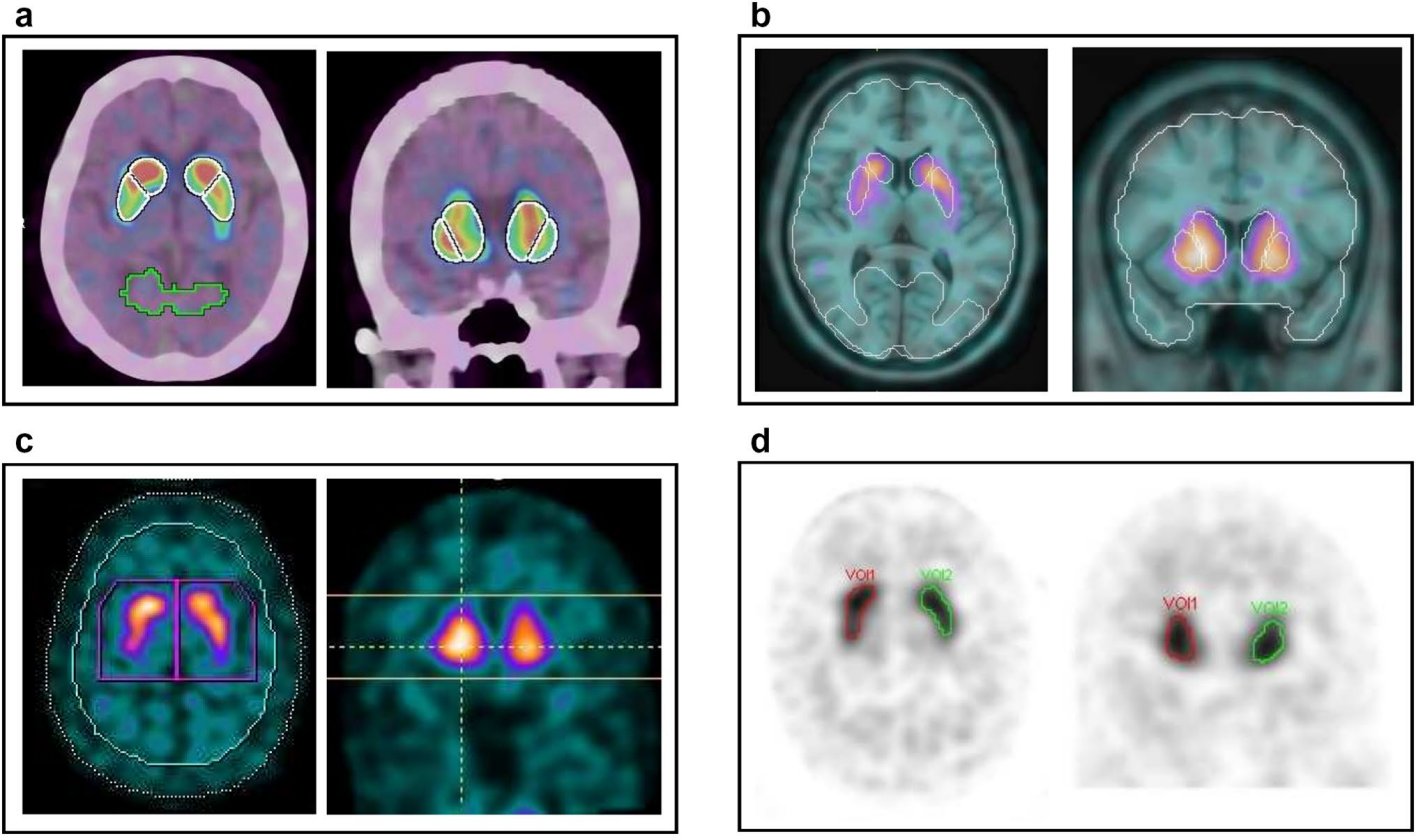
Outline of VOIs on striatal SPECT images using different methods for quantitative analysis. a DVR, b BR, c SBR, and d SUV
